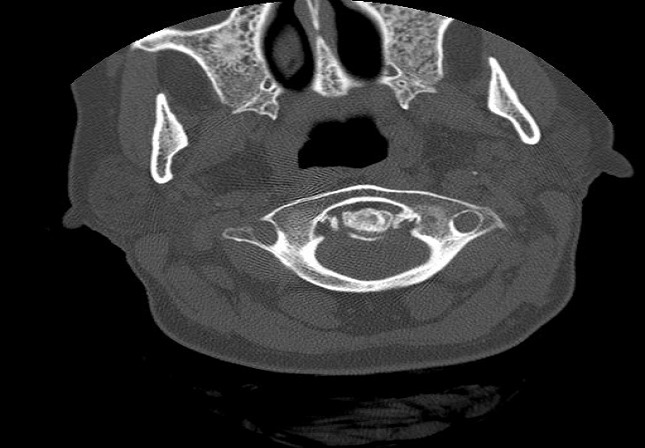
Axial computed tomography (CT) of the cervical spine revealing calcifications of transverse and alar ligaments, surrounding the odontoid process
